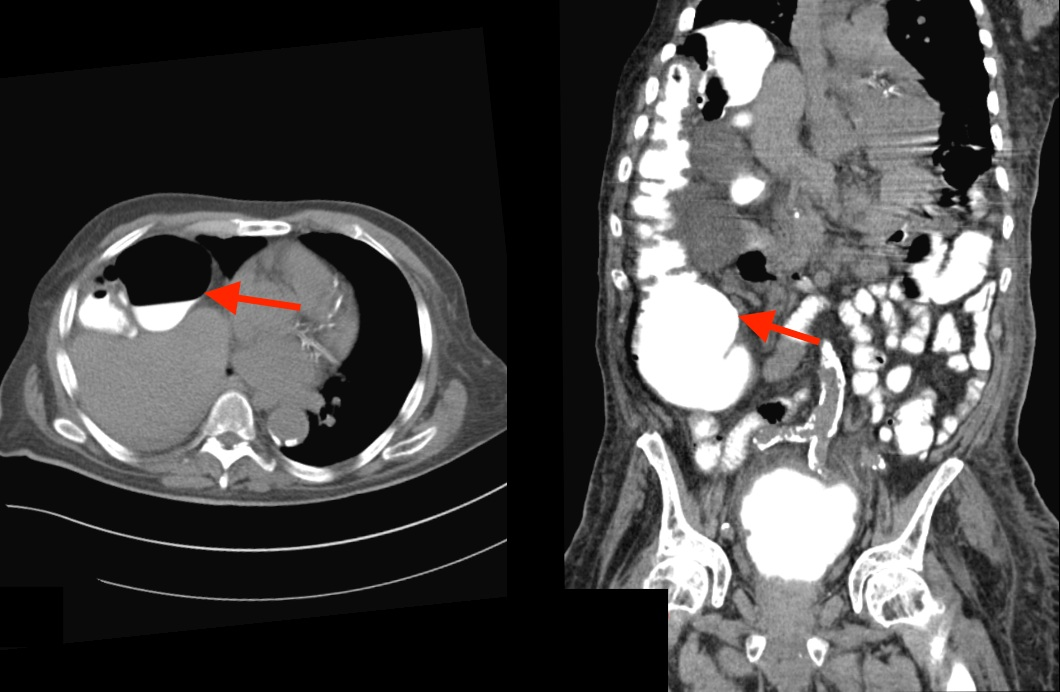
Abdominal computed tomography scan (axial and coronal views) with abdominal contrast showing colonic pseudo-obstruction.
